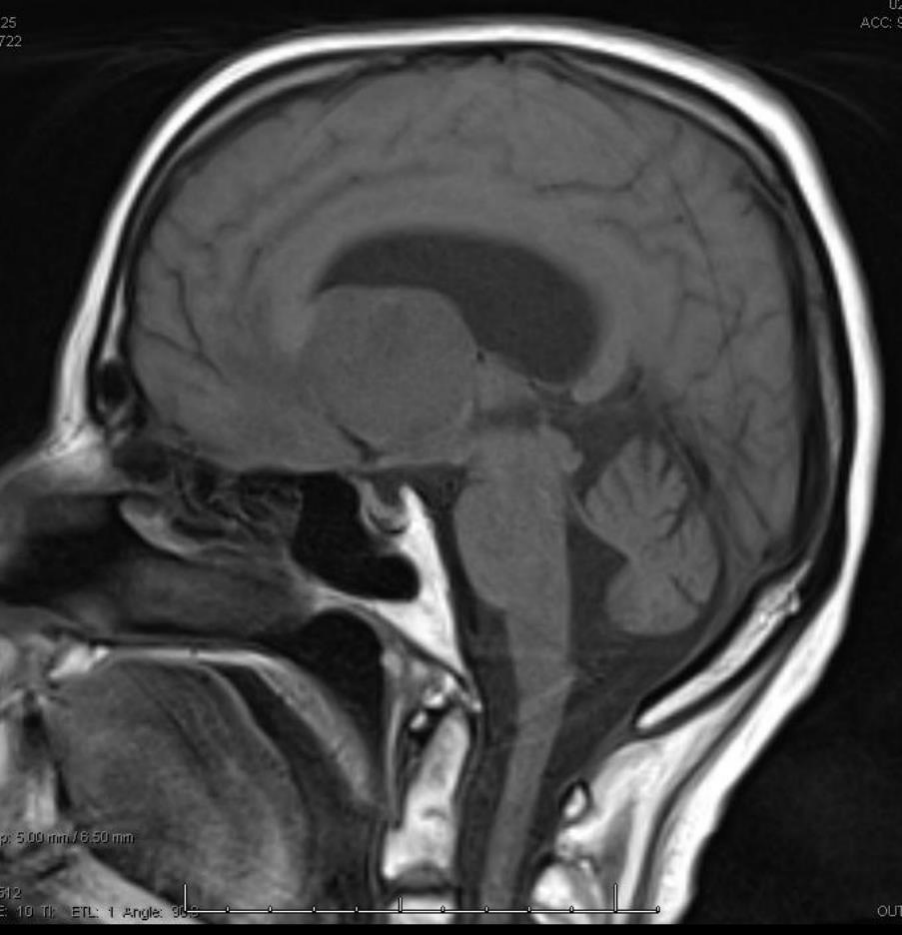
Initial MRI (pre-contrast) T1 sagittal pre-contrast MRI scan showing mass effect of the lesion on the foramen of Monro.
